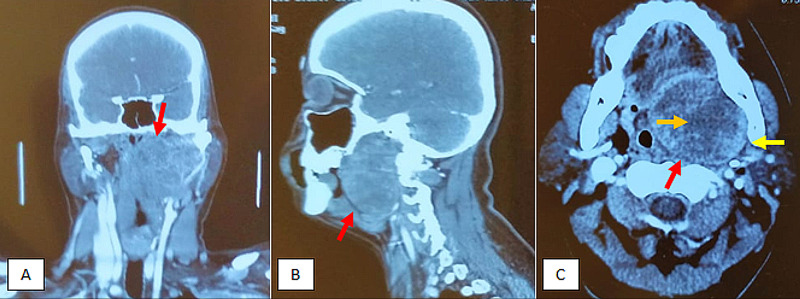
Computed tomography (CT) scan neck with contrast. (A): Coronal view of the CT scan depicting a mass in the parapharyngeal space that is superiorly abutting the base of the skull (red arrow). (B): Sagittal view of CT scan revealing the extension of the mass into the floor of the mouth (red arrow). (C): Axial view of the CT scan showing the involvement of the medial pterygoid muscle (yellow arrow), and extension of the mass into the prevertebral region (red arrow). There is also an area of internal necrosis (orange arrow).
